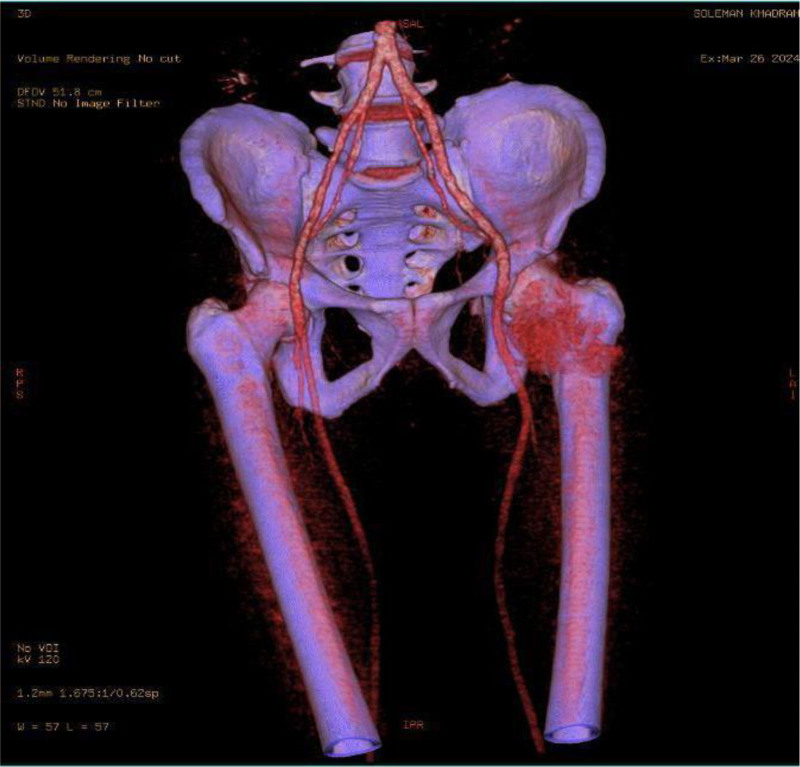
CT angiography revealing a highly vascularized tumor. CT = computed tomography.
